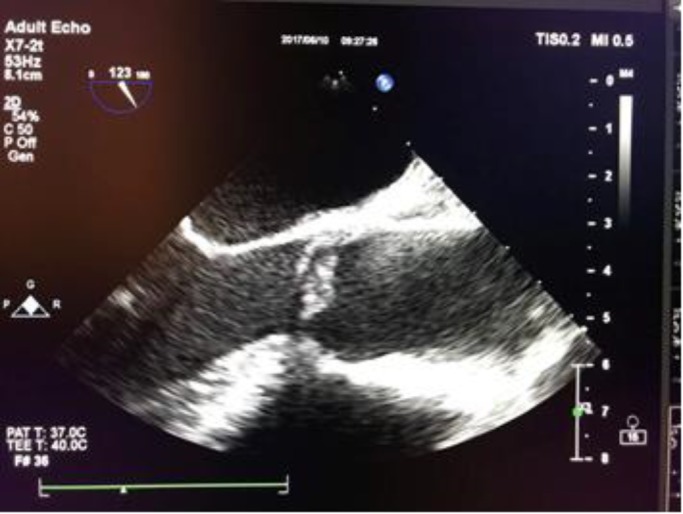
Transesophageal echocardiography (TEE) revealed a thickened mitral valve with two small vegetations on the tip of anterior (and posterior mitral valve leaflets
